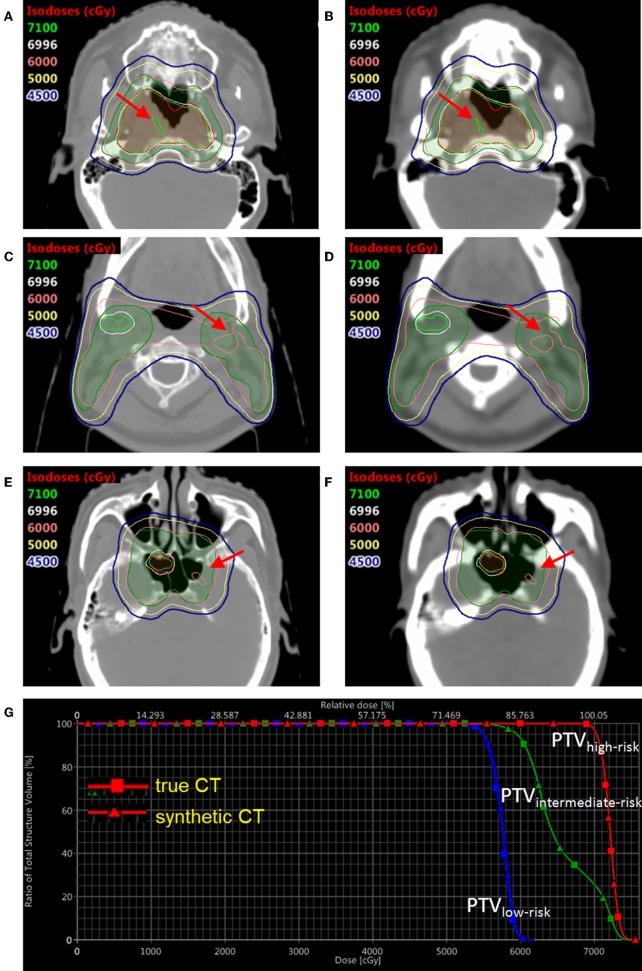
The dose distribution and DVH comparison between true CT and synthetic CT in an example NPC patient. Three slices of true CT are shown on the left (A,C,E); Three corresponding slices of synthetic CT are shown on the right (B,D,F). The DVH comparison based on true CT and synthetic CT is shown in (G). The translucent red region is high-risk PTV with prescription dose of 69.96 Gy; the translucent green region is intermediate-risk PTV with prescription dose of 60 Gy; the translucent blue region (not shown in axial views here) is low-risk PTV with prescription dose of 54.4 Gy. DVH with squares and triangles is based on true and synthetic CT, respectively.
